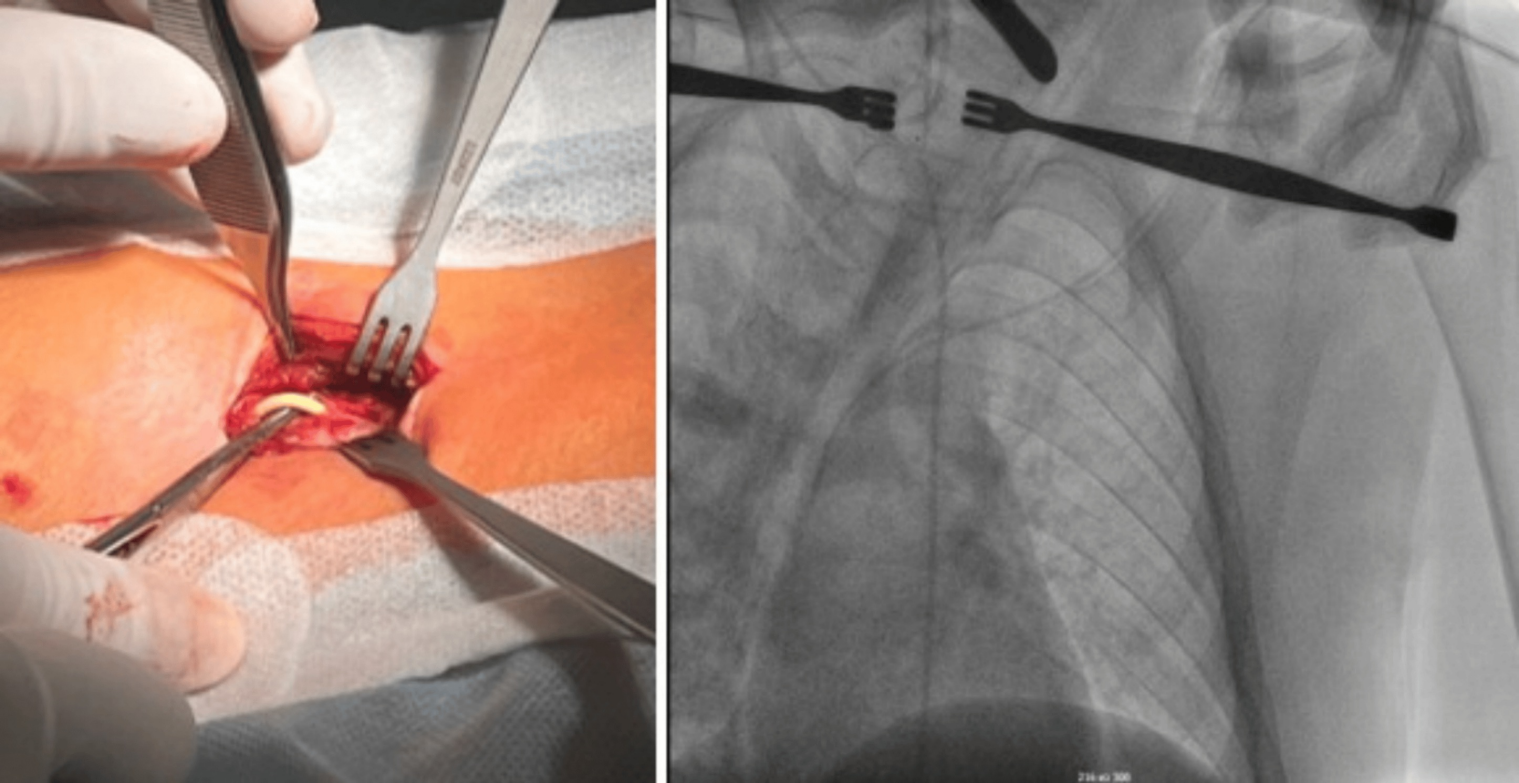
Interoperative photographs A: Incision on the neck and isolation of the peritoneal catheter from the soft tissue; B: Intraoperative X-ray control of catheter removal
